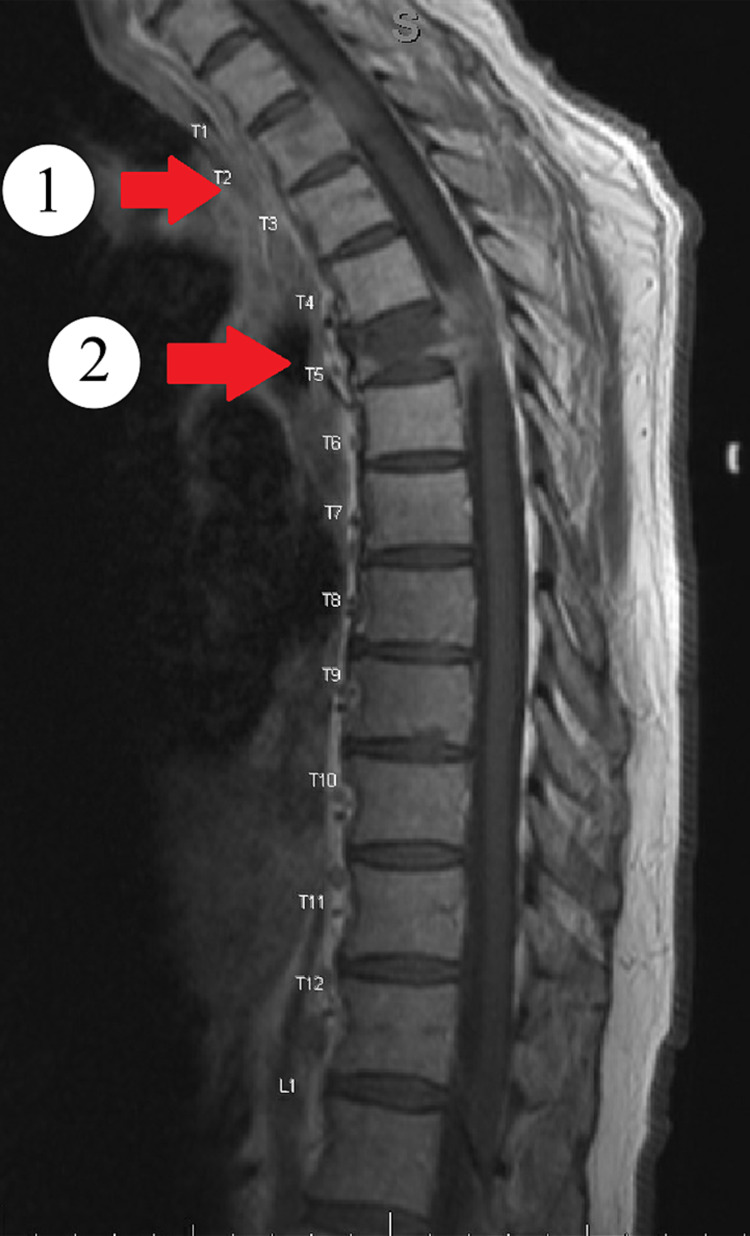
T1-weighted MRI image of the thoracic spine T1-weighted MRI image of the thoracic spine showing (1) an increasing retropulsion and epidural extraosseous metastasis causing moderate canal stenosis and cord compression. Increase in size of osseous metastatic lesion of the T2 vertebral body and left pedicle extending into the left neural foramina causing moderate left neural foraminal stenosis and (2) an increasing moderate to severe pathologic compression fracture deformity of T5 with approximately 75% height loss.
